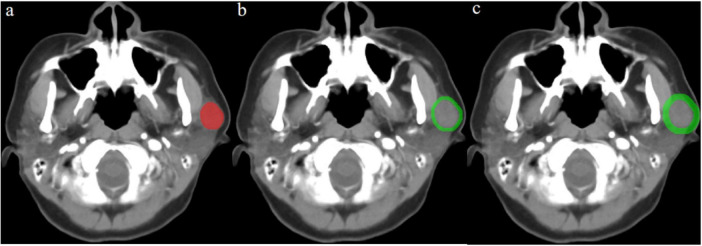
Contrast-enhanced computed tomography (CT) image from a pleomorphic adenoma patient, highlighted regions represent the primary tumor (a) and peritumoral region of 2 mm (b) and 5 mm (c).
